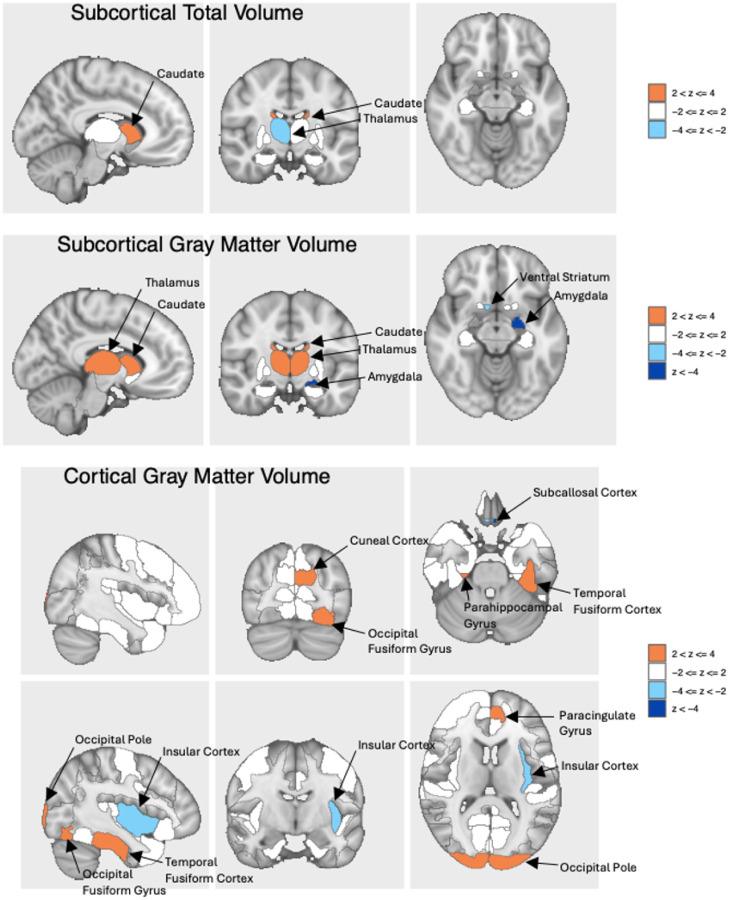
Partial results of BrainXcan association analysis for the second order externalizing + internalizing factor. Associations shown are for image-derived phenotypes from structural (T1) magnetic resonance imaging. Full results from BrainXcan are in Supplementary Figures 15–20 and Supplementary Tables 20–22. Blue colors represent reduced volume, and orange represents increased volume.
